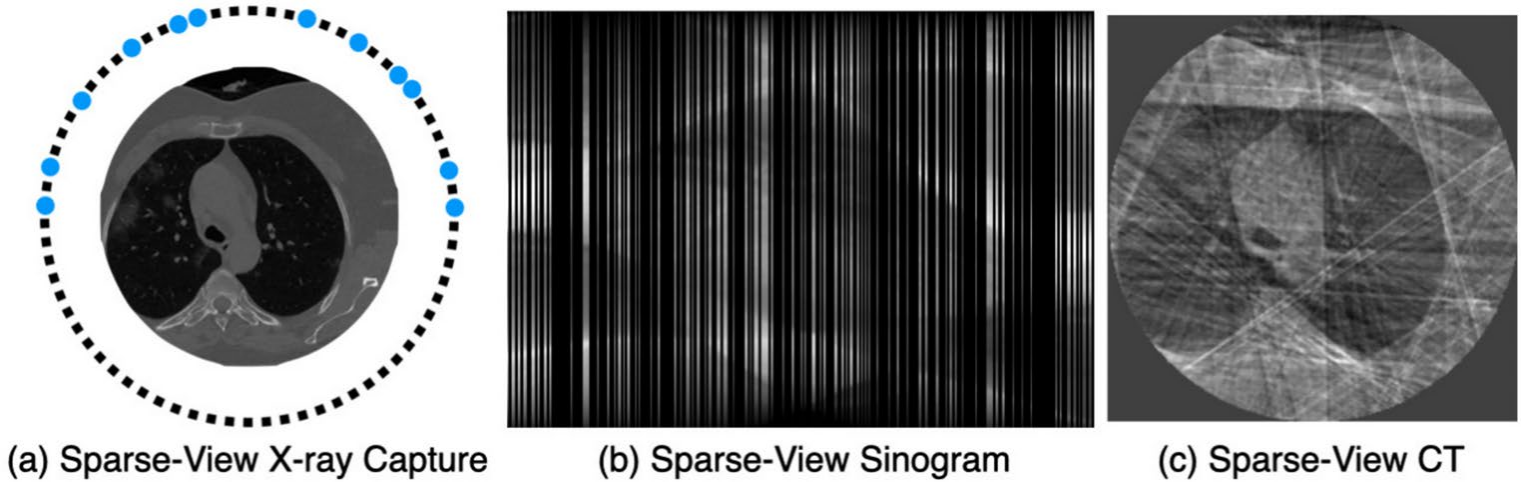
Randomly column-wise masking of the sinogram: The blue dots in (a) represent the points (angles) at which projections were acquired. These sparse projections form the sparse-view sinogram as shown in (b). This, in turn, is reconstructed to a low-quality CT image, as seen in (c), through Filtered Back Projection (FBP)
